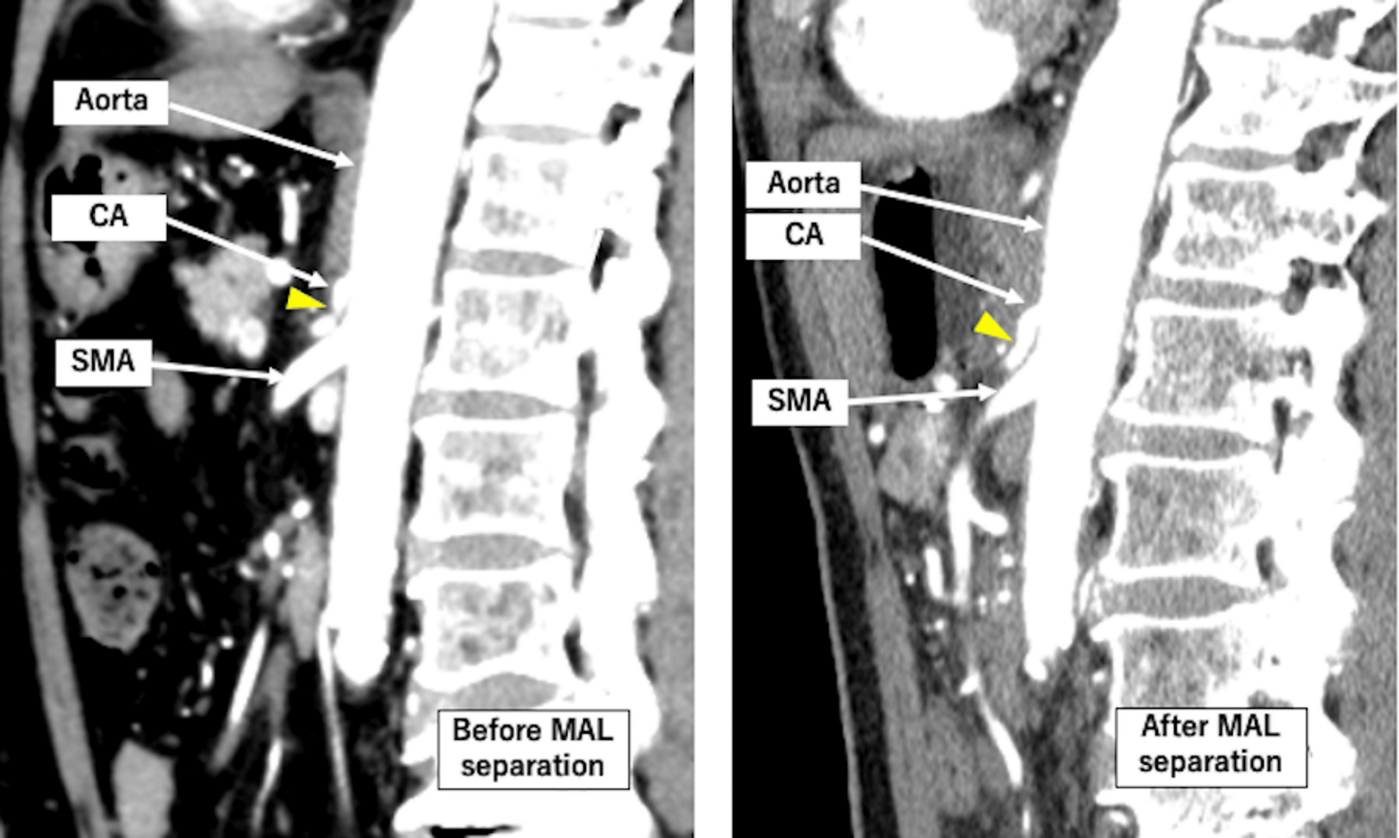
Contrast-enhanced CT of the thorax and abdomen. Sagittal section: (▲) CT images before and after MAL resection are shown. No calcification is present in the CA. (MAL: median arcuate ligament, CA: celiac artery, SMA: superior mesenteric artery)
